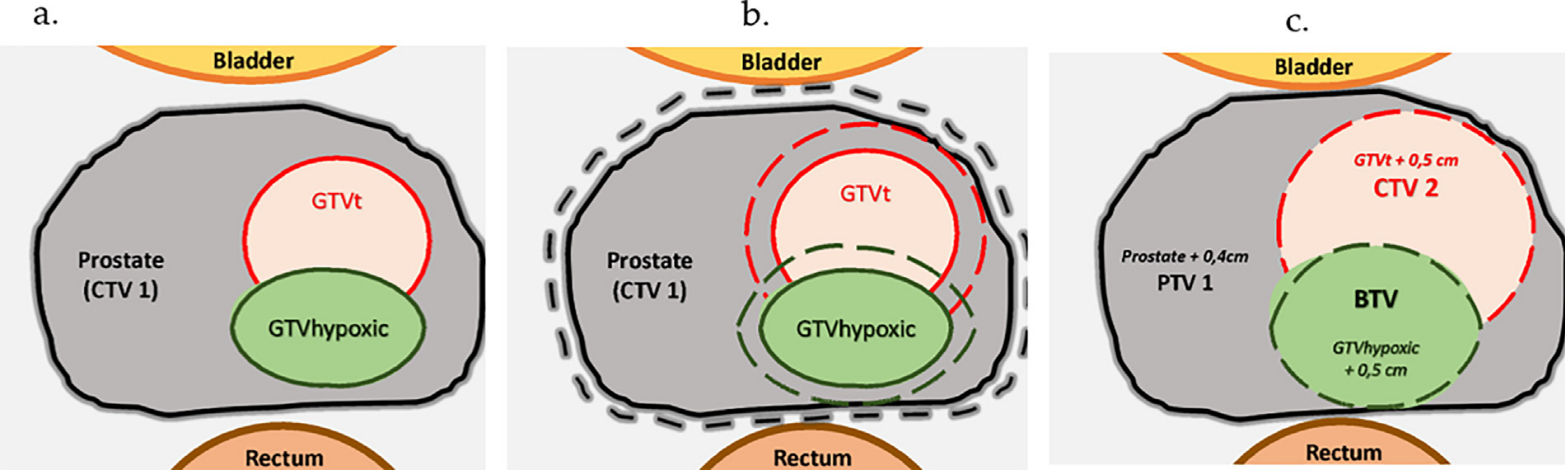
Representation of the target volumes gross tumoral volumes defined on magnetic resonance imaging (MRI) (target gross tumor volume [GTVt]) and on fluromisonodazole (F-MISO) (GTVhypoxic) (a), with an isotropic margin of 0.5 cm for the GTVt and GTVhypoxic and 0.4 cm for the whole prostate (clinical target volume [CTV1]) (b) to define the CTV2, biological target volume (BTV), and planned target volume (PTV1), respectively (c).
